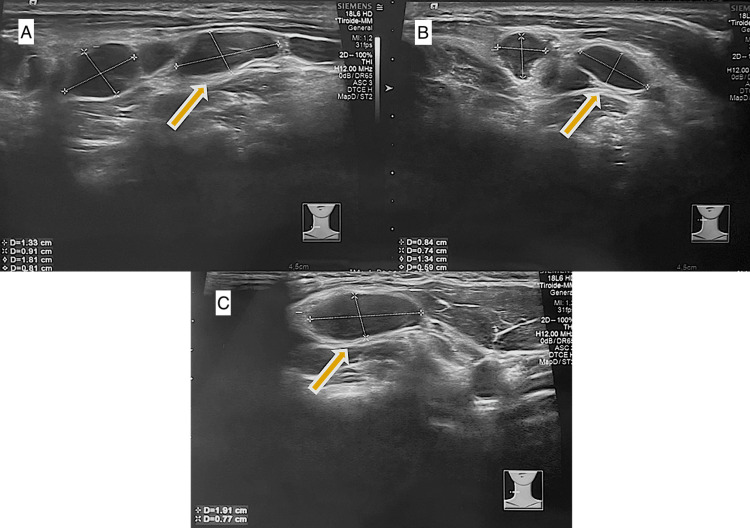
First ultrasound performed in Primary Healthcare Center First ultrasound performed in Primary Healthcare Center, showing various hypoechoic ganglion formations (A, B, C). Yellow arrow shows the largest ganglion formation measuring 19x7 mm.
